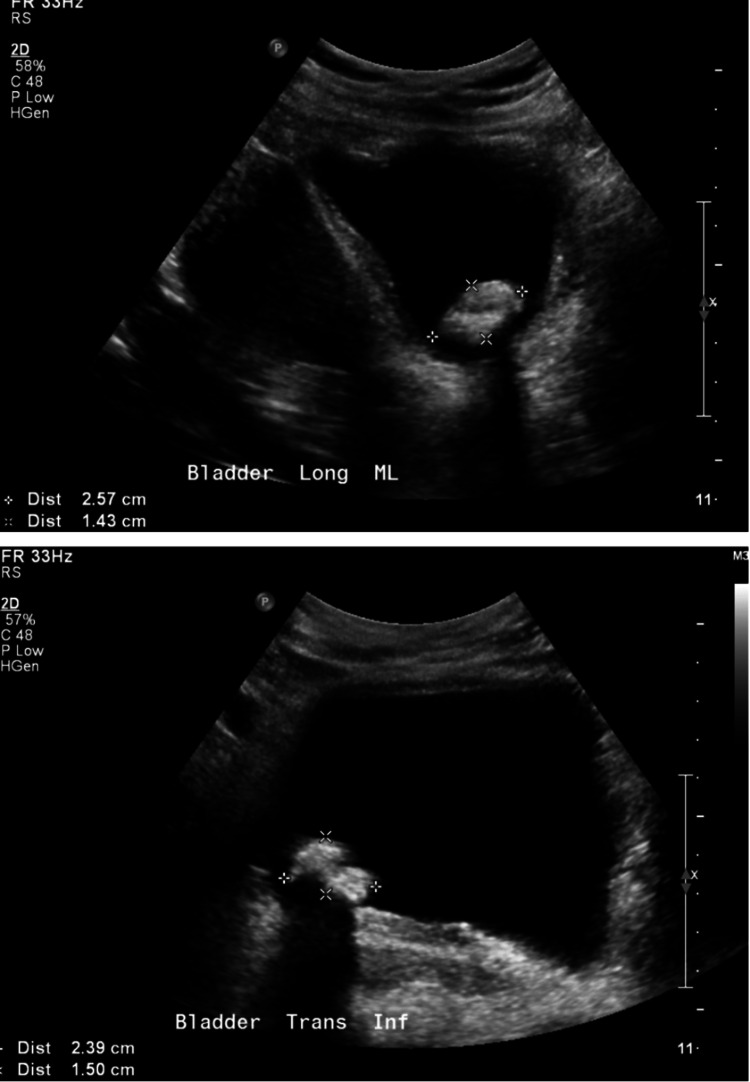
Pre-operative ultrasound further revealed the distally calcified ureteral stent in the urinary bladder
